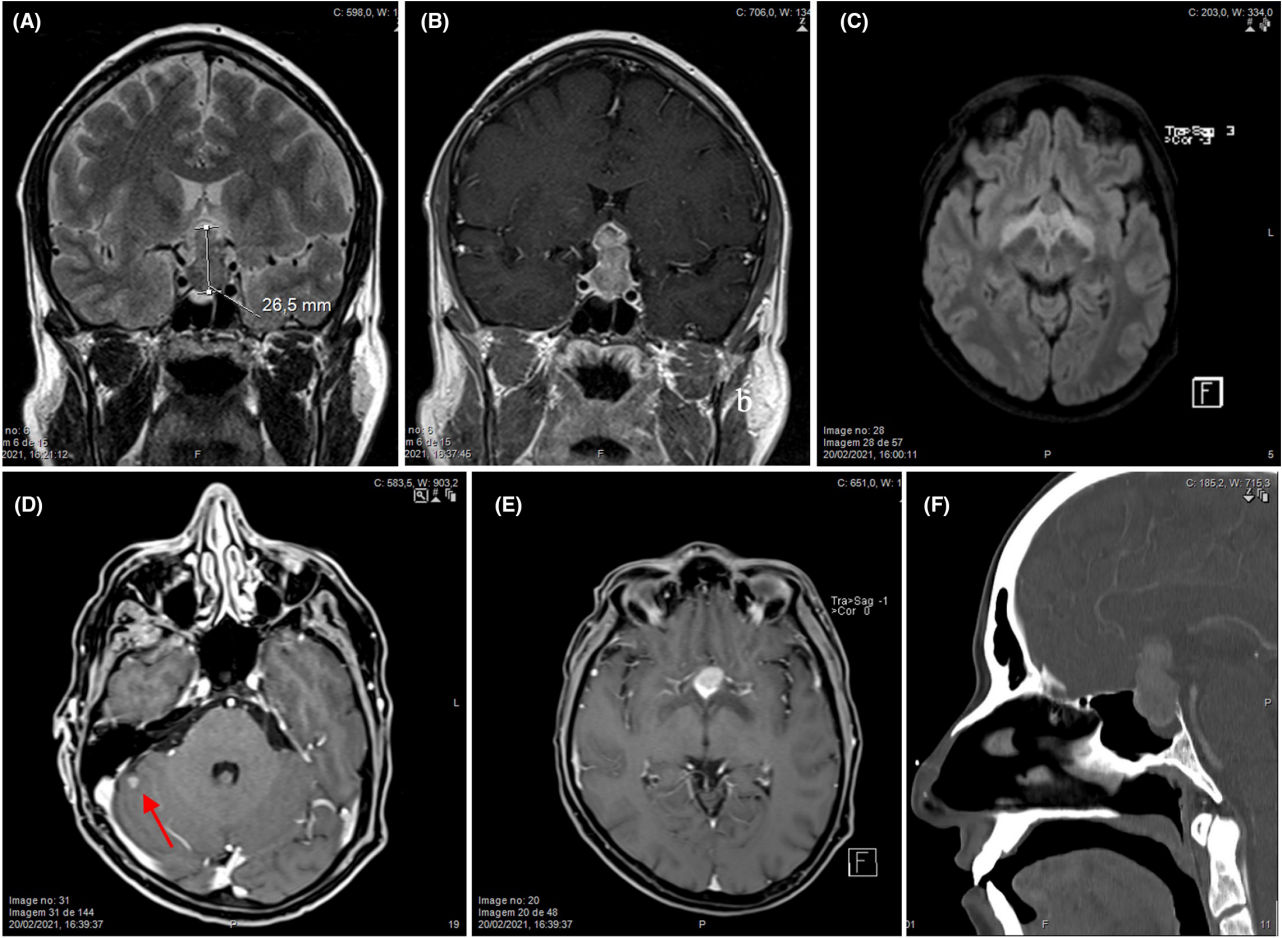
Coronal T2 (A) and T1 post‐gadolinium (B), axial T2 FLAIR reconstructed (C), axial and coronal T1 post‐gadolinium reconstructed (D, E) scans: 27 mm sellar and suprasellar lesion with marked superior shift of the optic chiasm and edema of the optic tracts and a 5 mm nodular enhancing lesion at the gray/white matter interface of the right cerebellar hemisphere (arrow, D). Sagittal CT section with post iodinated contrast medium (F) depicts erosion of sellar floor and dorsum indicating aggressive fast‐growing behavior.
